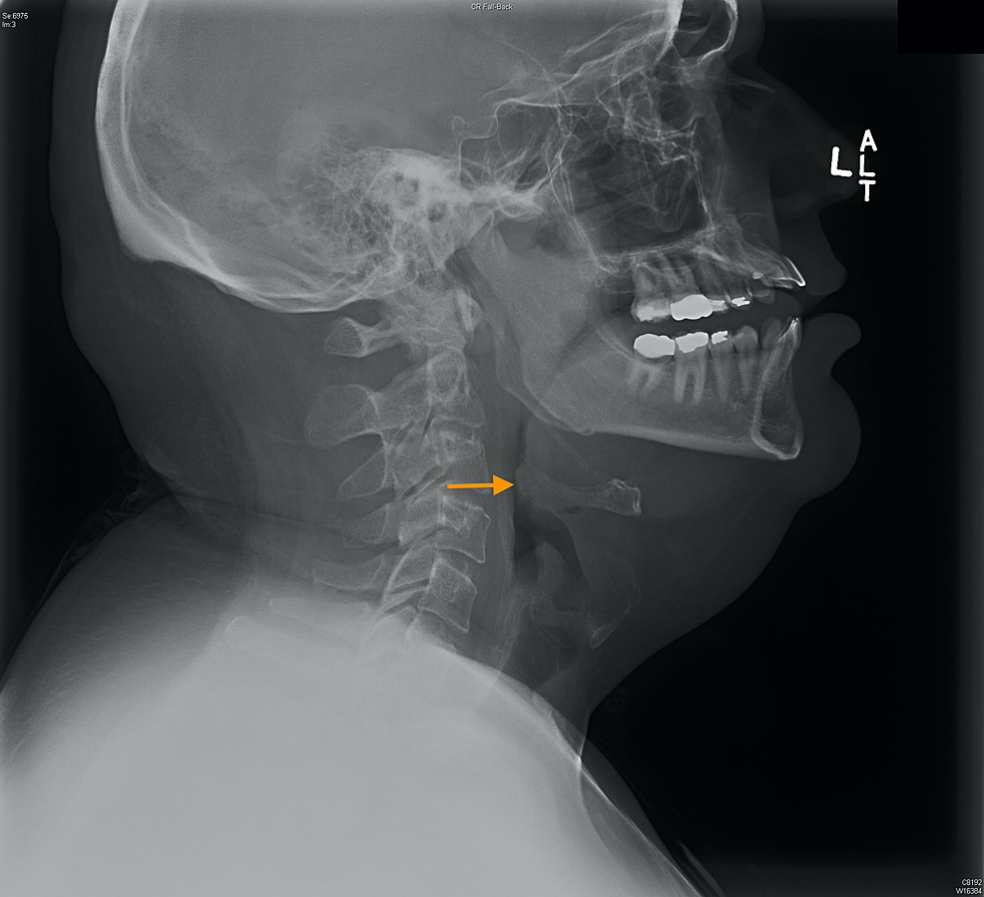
Lateral view radiograph of soft tissue of neck demonstrating severely narrowed airway (arrow)
